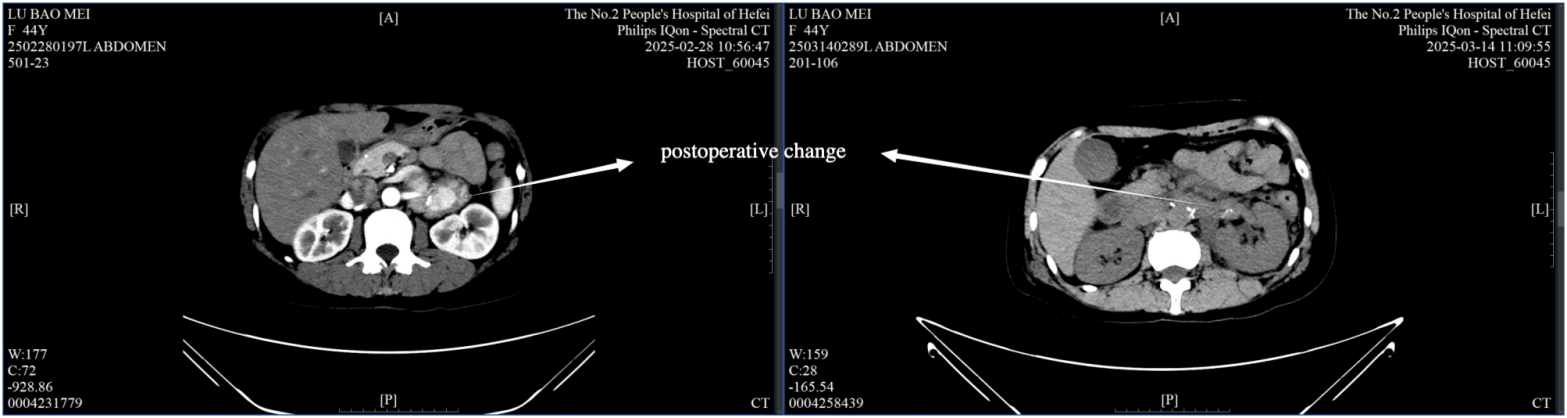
Postoperative non-enhanced CT scan.
